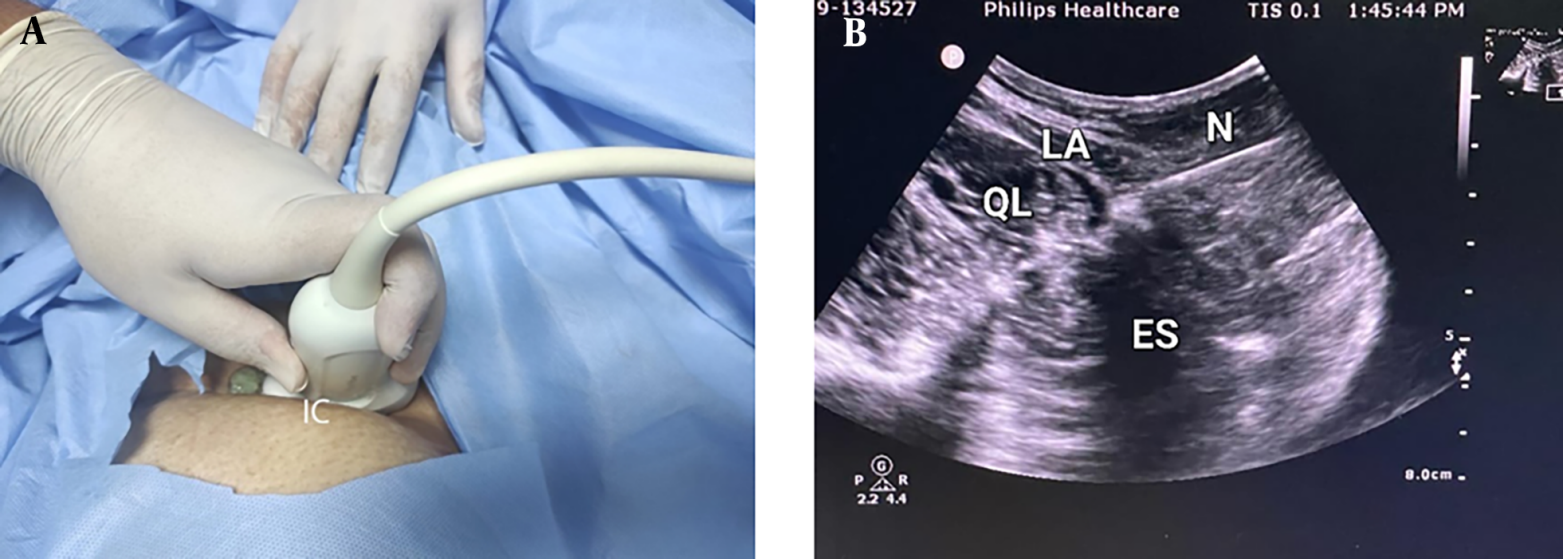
Quadratus lumborum block technique type II (A) position and (B) ultrasound image (IC, iliac crest; N, needle; QL, quadratus lumborum muscle).
